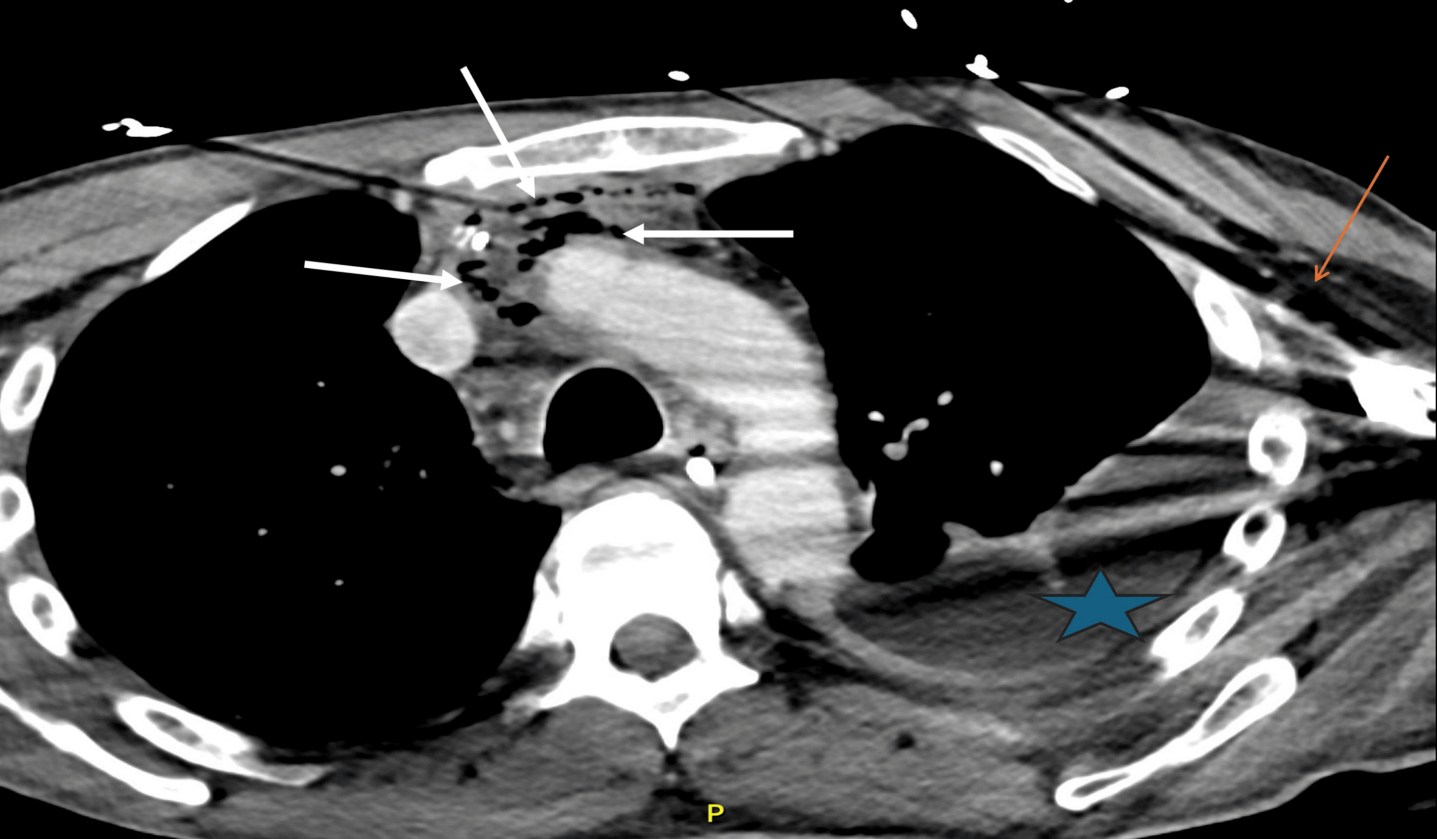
Follow-up axial CT scan demonstrating progressive necrotizing fasciitis and mediastinal involvement. Axial contrast-enhanced CT scan (two days post-initial surgery) demonstrating interval progression of necrotizing fasciitis. This view shows extensive gas foci (white arrows) within the anterior mediastinum and right upper chest, with new gas foci (red-orange arrow) in the left upper chest and axilla, along with a left pleural effusion (blue star). These findings indicate a persistent and spreading infection despite initial debridement.
